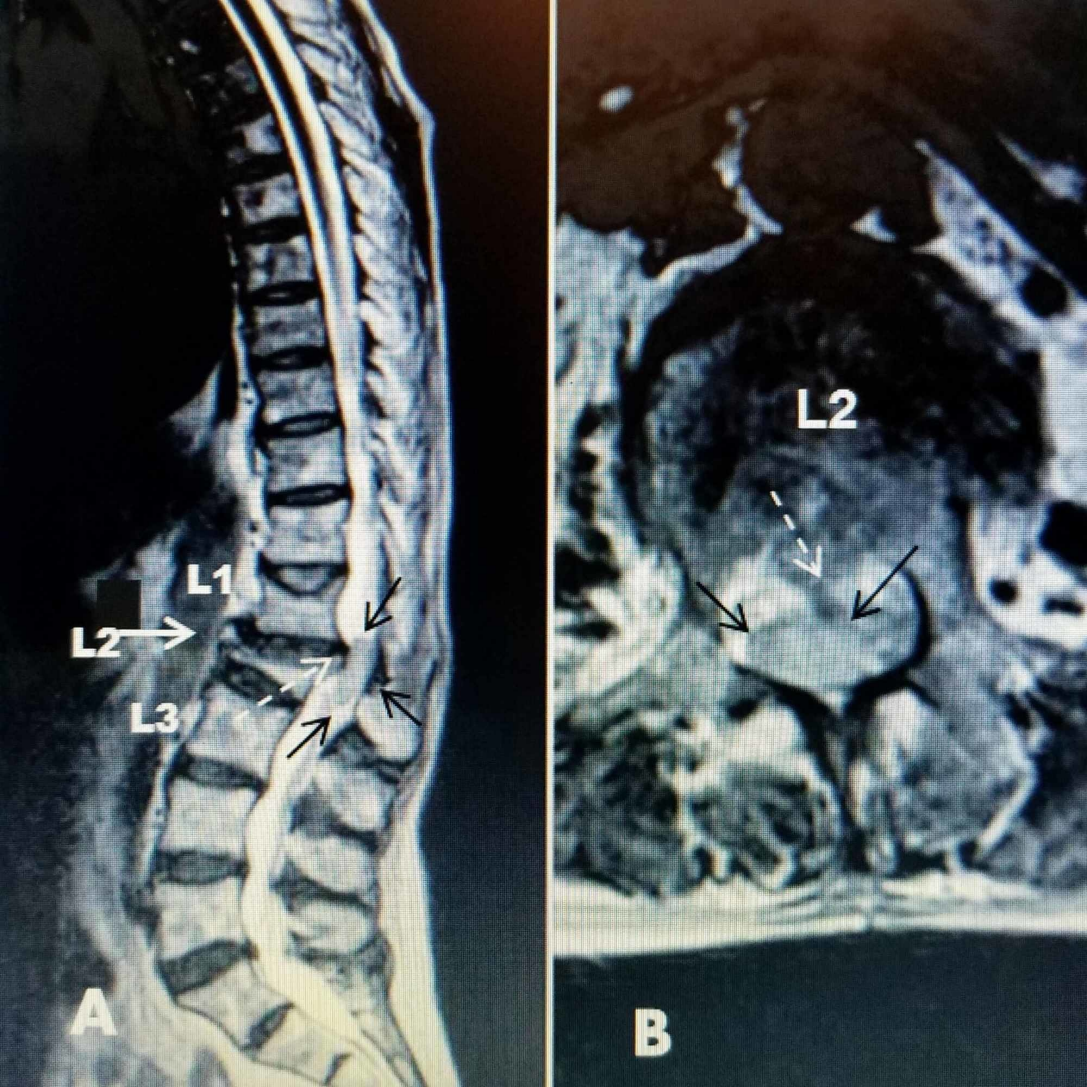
Initial MRI scan showing the L2 acute compression fracture and an intradural mass A: Sagittal spinal magnetic resonance imaging (MRI) showing an L2 70% wedge compression fracture (solid white arrow) with superior endplate edema and posterior displacement of 33% of the superior part of L2 (dashed white arrow) into the spinal canal. There is also minor superior endplate compression of L1. The isodense mass within the intradural space directly behind the L1-L2 disc space is clearly seen (solid black arrows) and, combined with the displaced endplate, causes almost 100% canal obstruction. B: Axial image at the level of the pedicles of L2 showing the homogeneous posterior intradural mass across the entire dorsal half of the spinal canal (solid black arrows). No conus or nerve roots are identified. The midline dorsally displaced posterior edge of L2 is seen just ventral to the intradural mass but comprising less than 50% of the axial compression (dashed white arrow).
